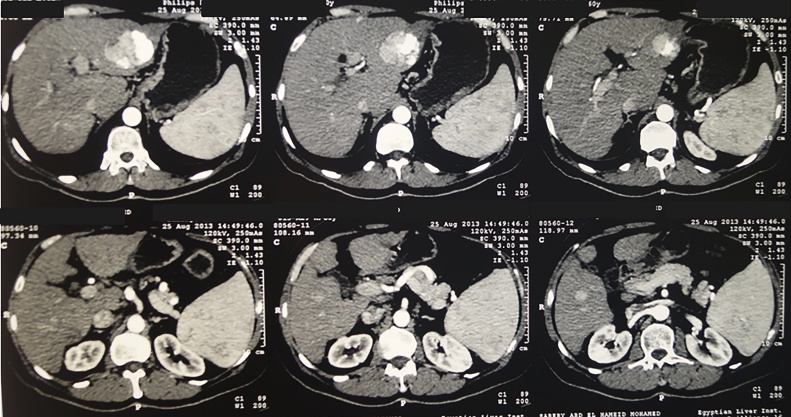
Abdominal computed tomography before liver transplantation showing large left hemi-liver hepatocellular carcinoma with partial lipidol uptake and other smaller ones in both hemi-livers.
